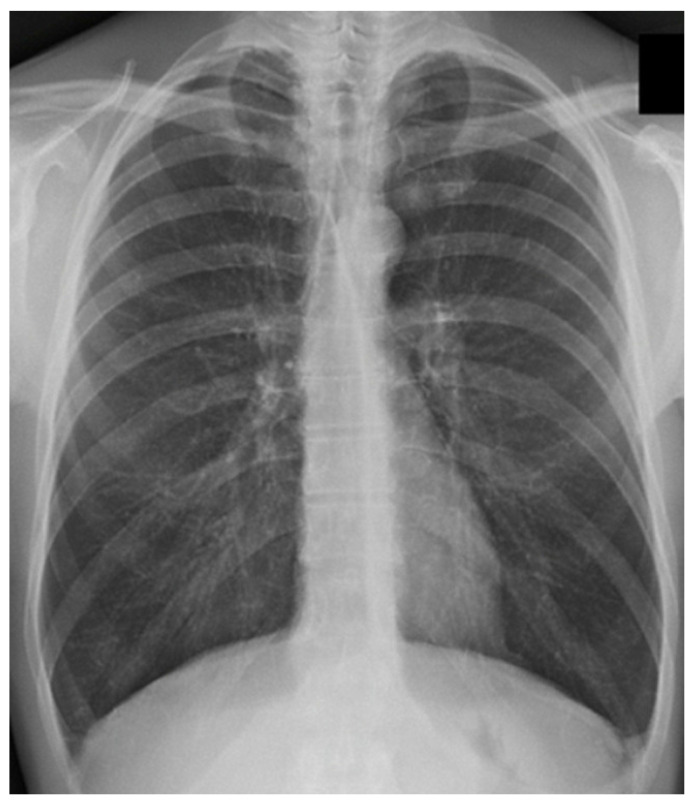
Chest x-ray of a COPD patient. The diaphragm is in a chronic inspiratory position (lung hyperinflation or hyperdistension) with a more flattened morphology. The constant, non-physiological position of the hemi-cupules causes a reduced elasticity of the lung parenchyma, an adaptation that parallels the chronicity of the disease. The photo was taken by Bruno Bordoni.
